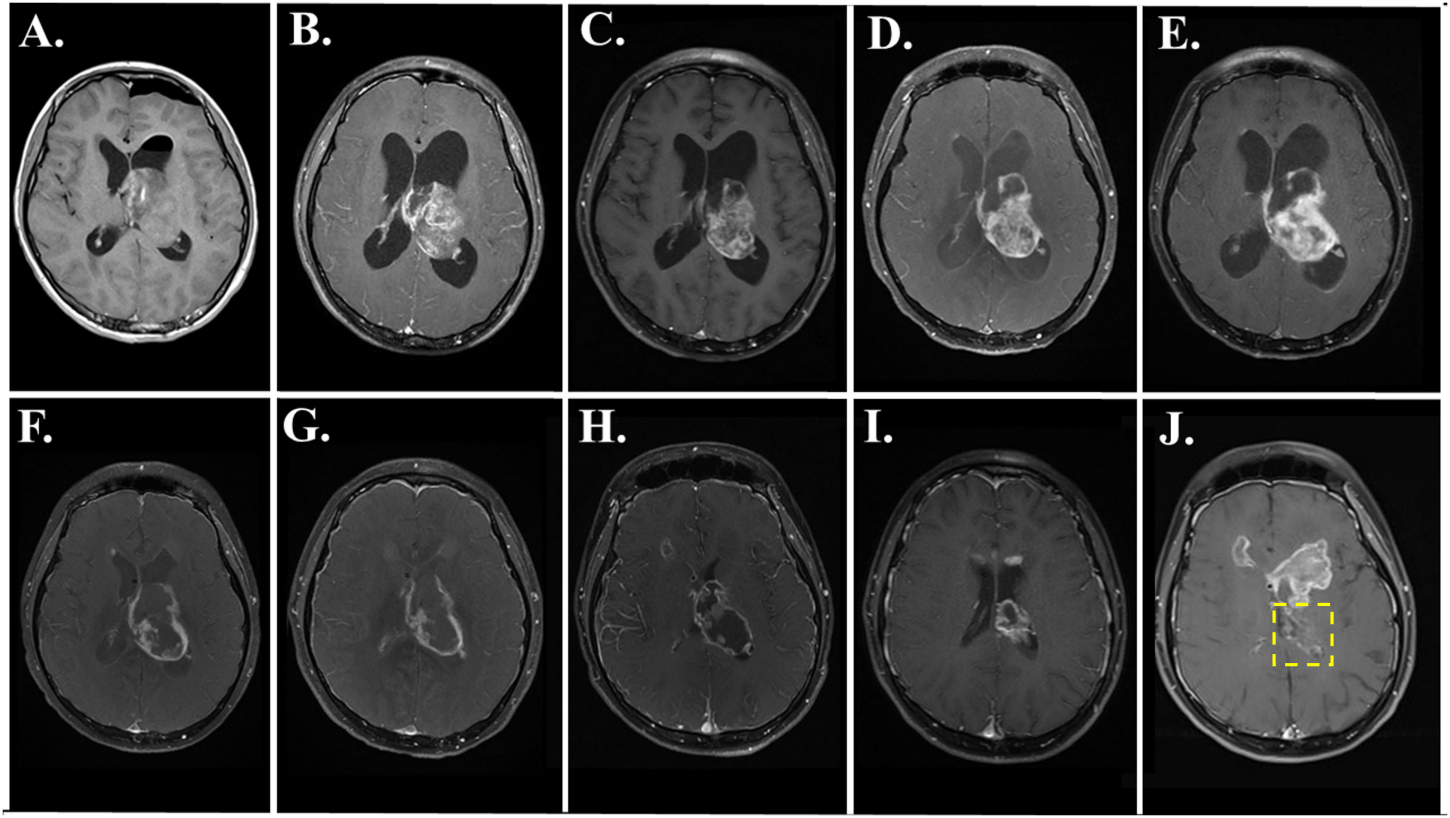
MRI images showing primary DMG tumor regression during and after FPM therapy with disulfiram and ONC201. T1-post gadolinium axial images are shown from the following days: (A) day 2; (B) day 88; (C) day 149; (D) day 203; (E) day 225; (F) day 255; (G) day 288; (H) day 351; (I) day 409; and (J) day 469. In J, the location of the primary tumor is within the yellow box, and secondary tumors are evident above the location of the primary tumor.
